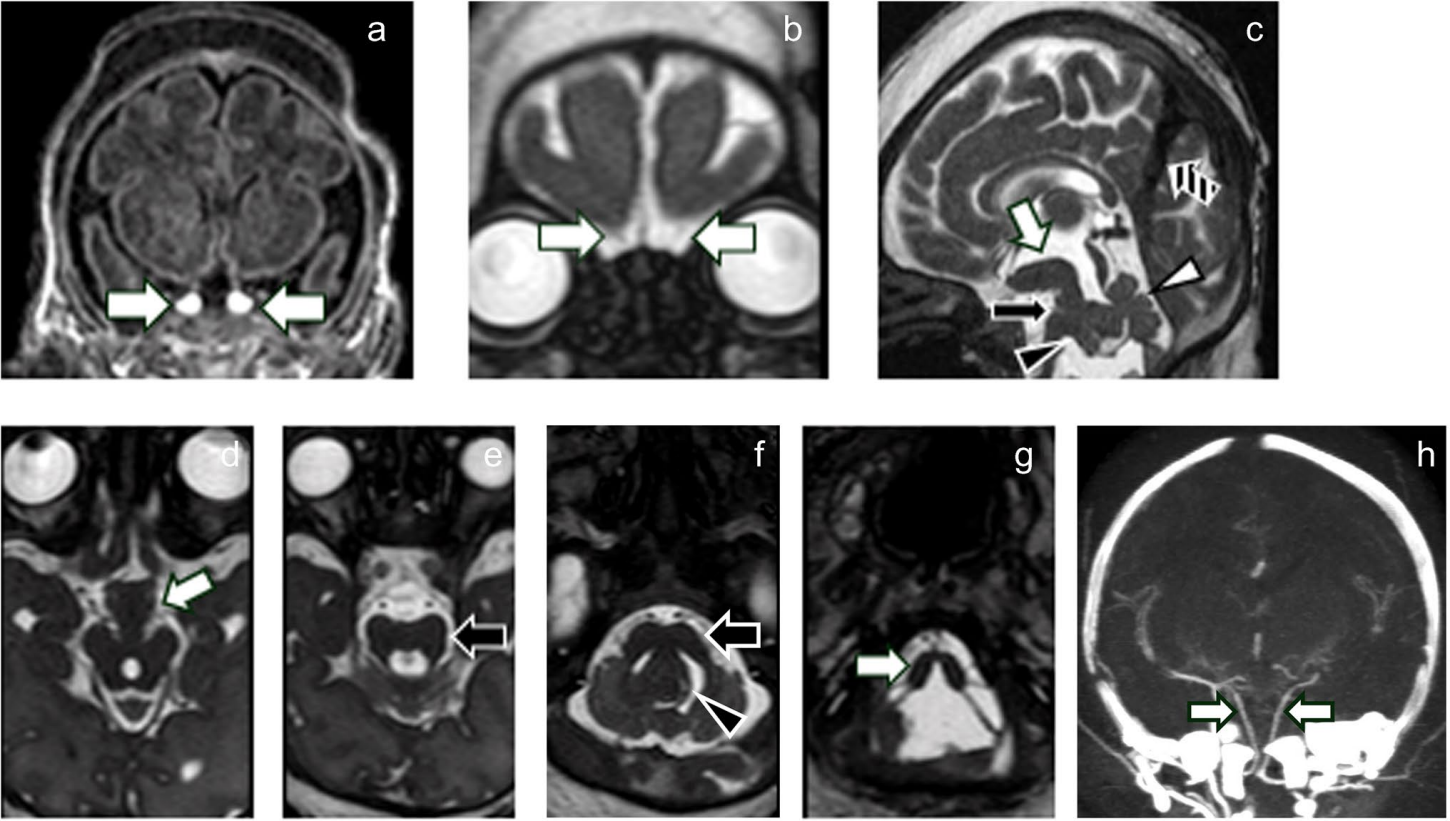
Intracranial malformations. Coronal T1-weighted MRI (a) demonstrates duplication of the pituitary gland and infundibulum (*arrows* in a). Coronal balanced fast-field echo T2-weighted MRI (b) demonstrates marked olfactory bulb hypoplasia or aplasia (*arrows*). Sagittal (c) and axial (superior to inferior, d-g) balanced fast-field echo T2-weighted MRI demonstrates a “prominent hypothalamic midline” and diencephalic-mesencephalic junction abnormality (incomplete separation of the diencephalic tissue and midbrain; *white arrows* in c and d). There is an unusually severe hindbrain malformation including a widened pons with narrow AP diameter (*black arrows* in c, e, and f) and subtle ventral clefting (*black arrow* in c), cerebellar hemispheric hypoplasia, vermian hypodysplasia with increased tegmentovermian angle (*white arrowhead* in c), dysplastic tissue resembling the cerebellum that is inseparable from the dorsal pons and lateral aspect of the fourth ventricle (*black arrowheads* in c and f), small middle cerebellar peduncles, and an abnormal-appearing medulla with a deep dorsal cleft (*white arrow* in g). There is a persistent falcine sinus (*striped arrow* in c). Non-fused paired vertebral arteries are seen on a coronal CT angiogram maximum intensity projection image (h).
